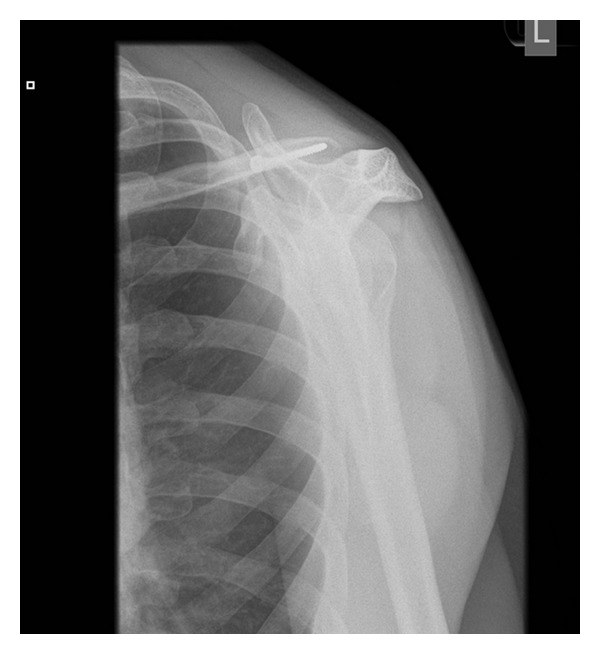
Postoperative Y view radiograph showing the reduced joint with screw in situ.
